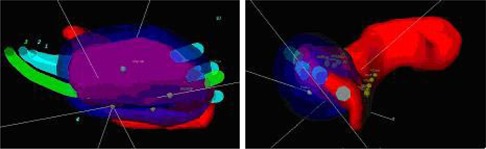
(a) Lateral and (b) posterior view of the reconstructed stent and 100% isodose cloud for the CT‐based plan
